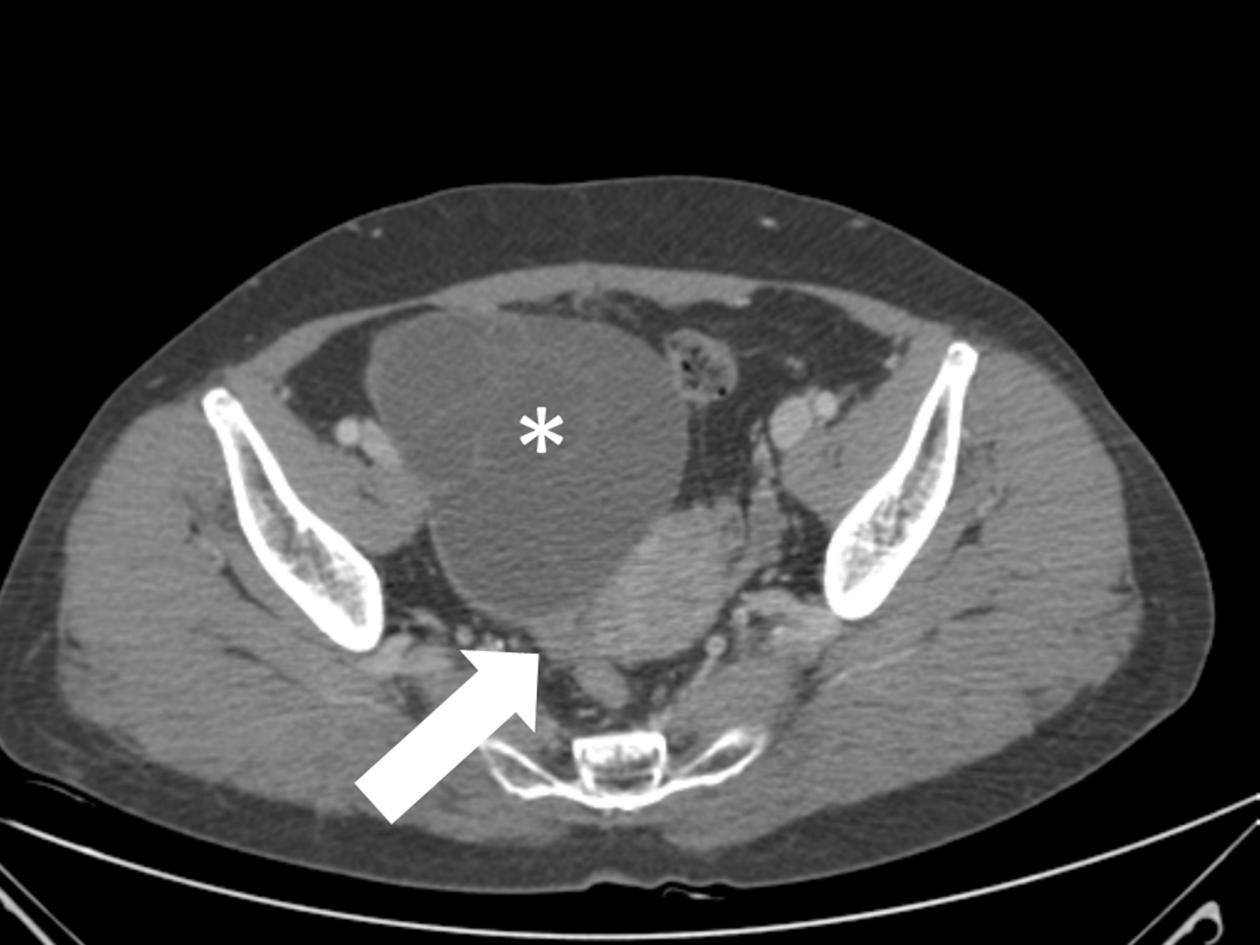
A 58-year-old woman with right ovarian mucinous cystadenoma. Axial contrast-enhanced CT scan shows a thickened pedicle (arrow) between a right ovarian cystic mass (asterisk) and the right uterine cornu. The thickened pedicle is not associated with a whirls sign or a navel sign. Uterine deviation is also not noted (not seen here). Surgery revealed a right ovarian cystic mass without torsion.
